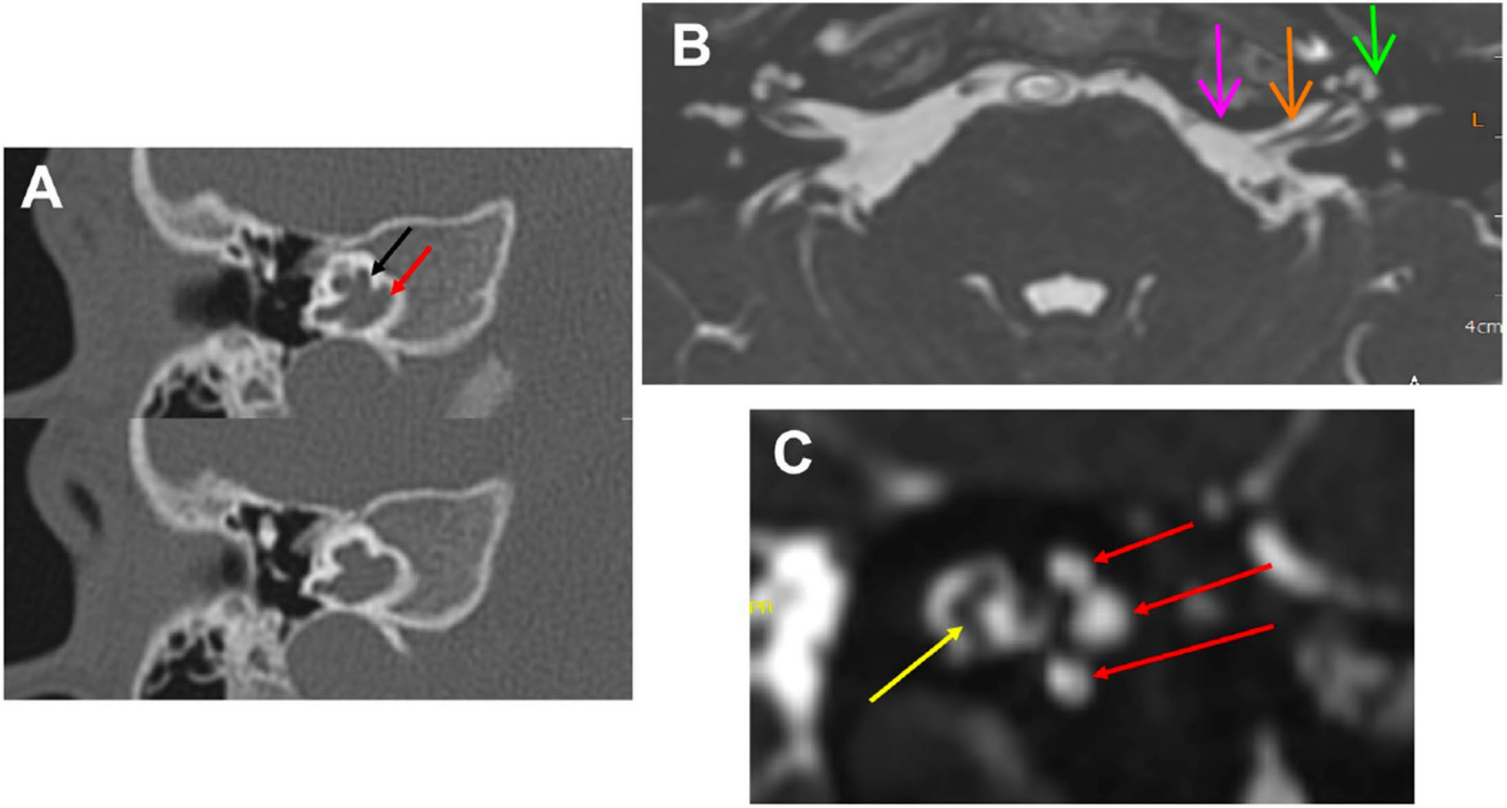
MRI and Inner Ear: (A) Computed tomography of the right temporal bone in a patient with of X-linked deafness. It shows an open connection between the CSF space and the perilymphatic space. The black arrow points to the basal turn of the cochlea and the red arrow points to the internal auditory canal. This is one possible malformation with a connection between these spaces. Others would be cases with a missing modiolus or defects in the modiolus itself. (B) MRI axial view of the brain, root entry zone of cranial nerve VIII (pink arrow), internal auditory canal (orange arrow), and inner ear (green arrow). The CSF (in white) goes through the internal auditory canal into the cochlea and vestibule (inner ear). (C) MRI parasagittal view of the internal auditory canal contains cochlear and vestibular nerves (yellow arrow) and CSF (in white); which infiltrates all three turns of the cochlea (red arrows).
